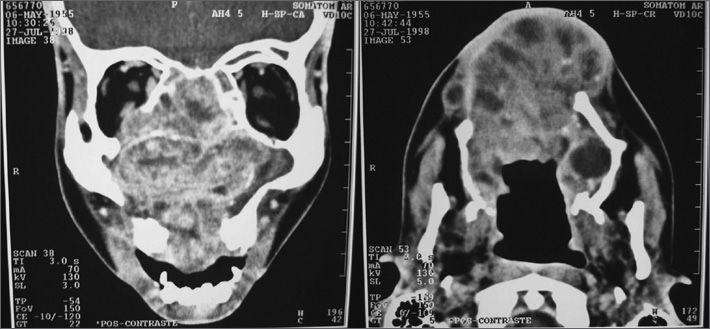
CT scan showing a large solid mass occupying part of the right-side maxillary sinus, palate and nasal cavity.
